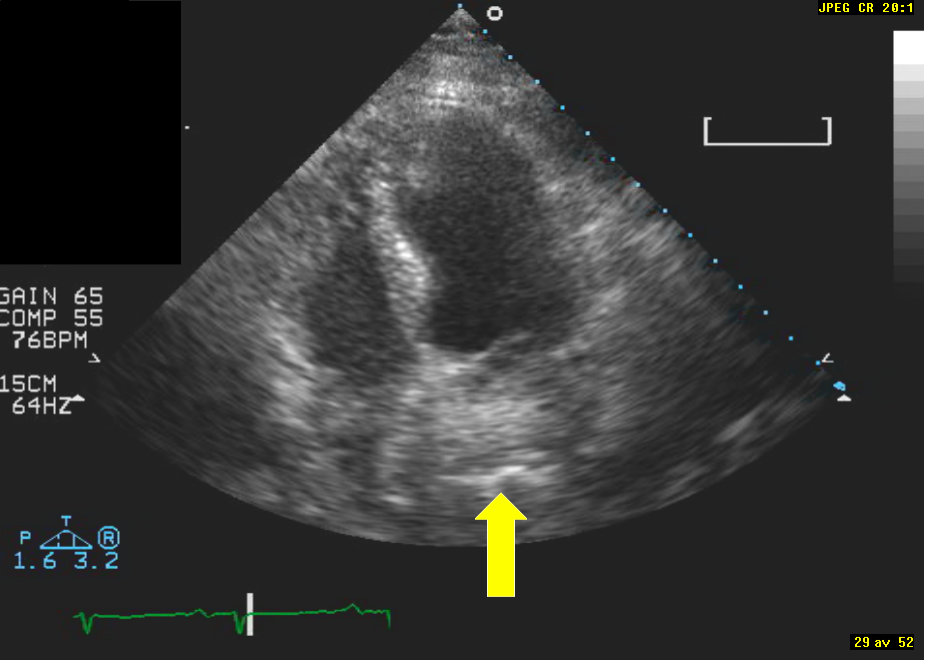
Image from apical 4-chamber view. The arrow indicates the hiatal hernia.
